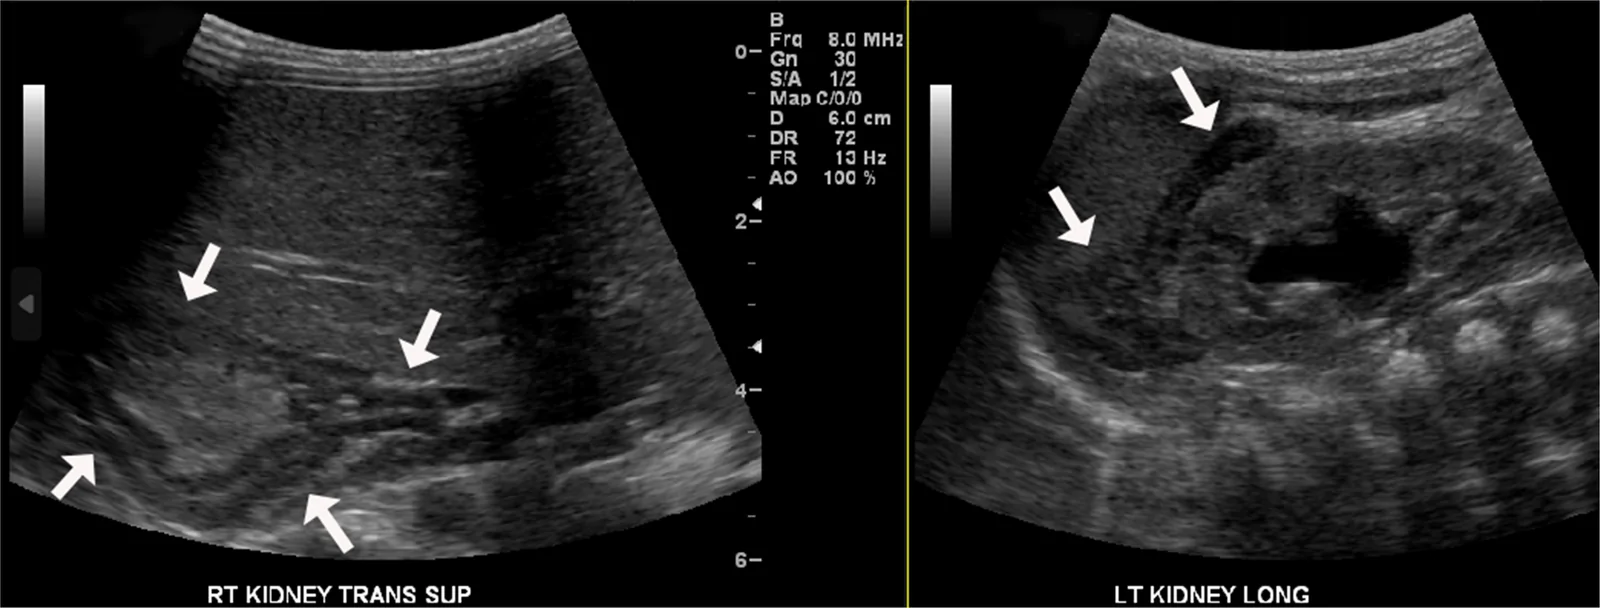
Congenital adrenal hyperplasia due to 3-beta hydroxysteroid dehydrogenase deficiency in a 4-day-old male infant imaged by ultrasound. Adrenal glands shown in transverse and longitudinal planes are larger than expected size of the adrenal glands relative to the kidney and also have a slight cerebriform morphology (*arrows*)
